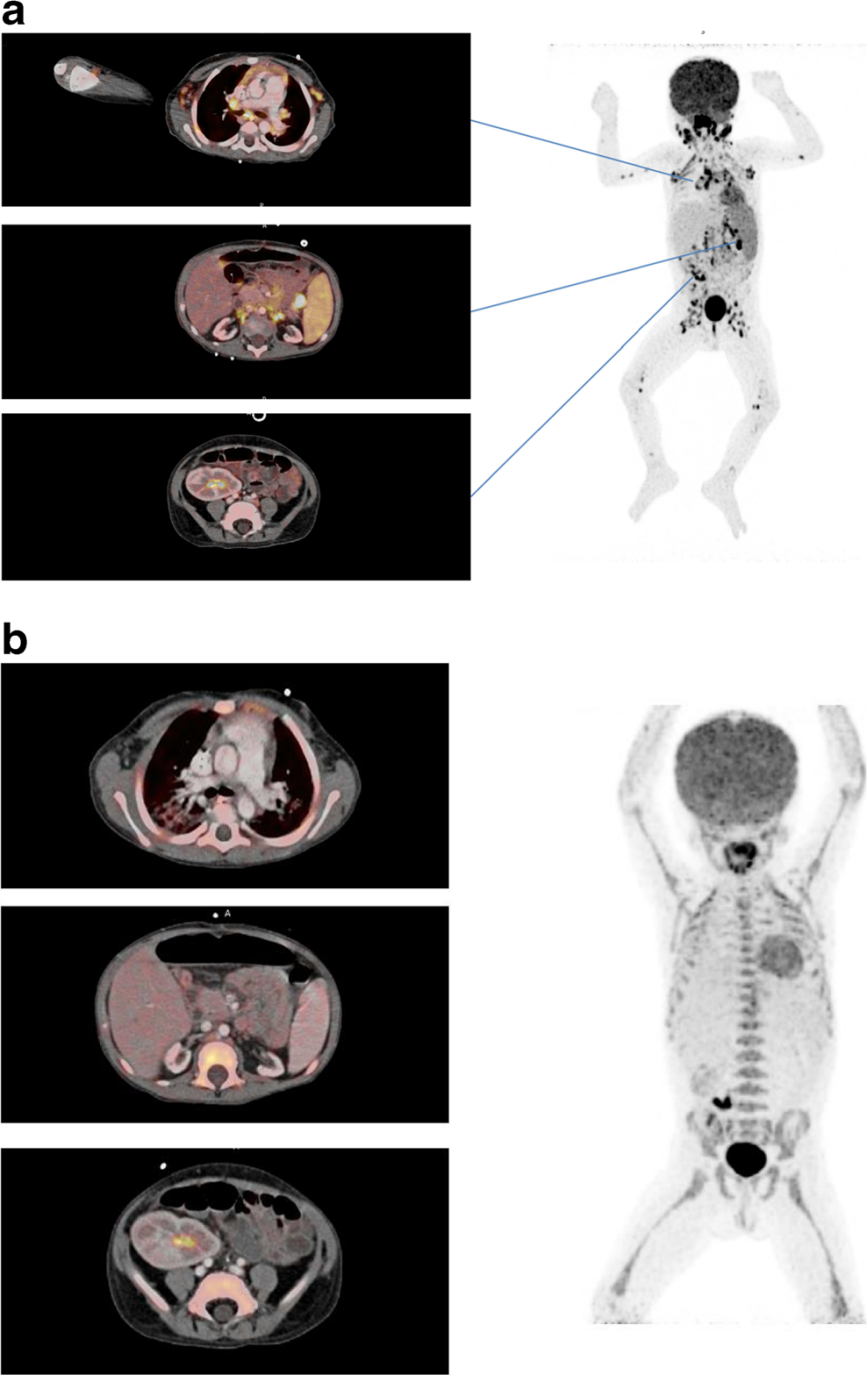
A three year old kidney and liver recipient with fever and elevated EBV DNA in plasma 3 months after transplantation, suspected for post-transplant lymphoproliferative disorders (PTLD). A) The FDG PET/CT showed increased FDG uptake in enlarged and normal sized lymph nodes above and below diaphragm including the extremities, in the rhinopharynx, tonsils, and spleen compatible with post-transplant lymphoproliferative disorders. B) Complete metabolic and structural remission after 3 weeks of rituximab treatment. The area with increased FDG-retention in the right fossa corresponds to urine excretion in the well-functioning graft
